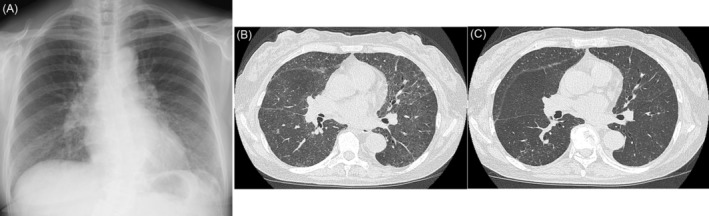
(A) Chest x‐ray showing the ground‐glass opacities in the bilateral lung fields. (B) Thoracic computed tomography (CT) obtained upon admission showing bilateral diffuse nonsegmental ground‐glass opacities. (C) CT obtained 14 days after admission showing improvement of ground‐glass opacities in the bilateral lung fields.
